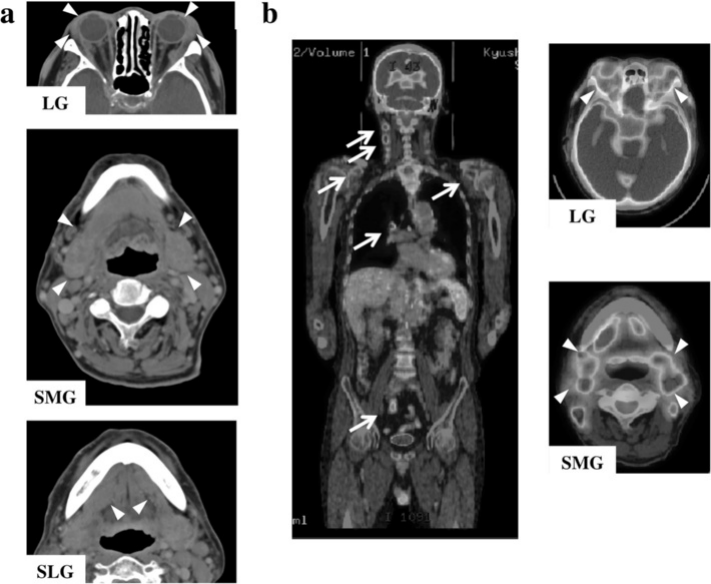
Imaging findings before treatment. a CT findings indicating swelling of LGs, SMGs and SLGs (*white arrowheads*). b FDG-PET indicating abnormal multiple accumulations in SMGs and LGs (*white arrowheads*) and in multiple systemic lymph nodes (*white arrows*)
